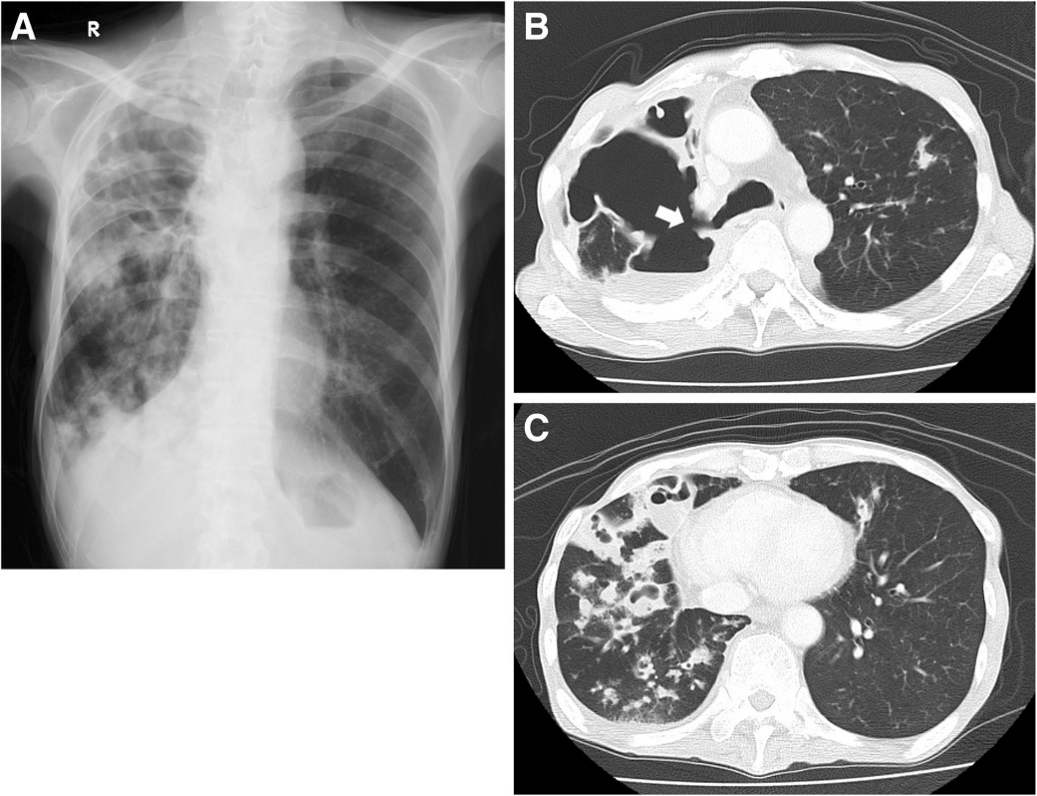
Radiological examination on final admission. (A) Chest radiograph showing an enlarged air space in the right upper lung field and a diffuse infiltrative shadow. (B, C) CT images demonstrating a large air space with niveau formation and multiple cavitary lesions in the residual lung, respectively. A right upper bronchial fistula (arrow) can be seen.
